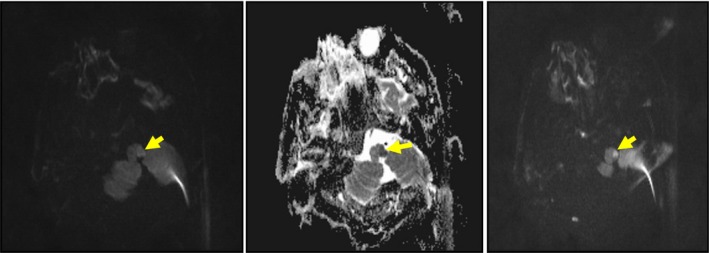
DWI and ADC left bulbar acute ischemic lesion.
